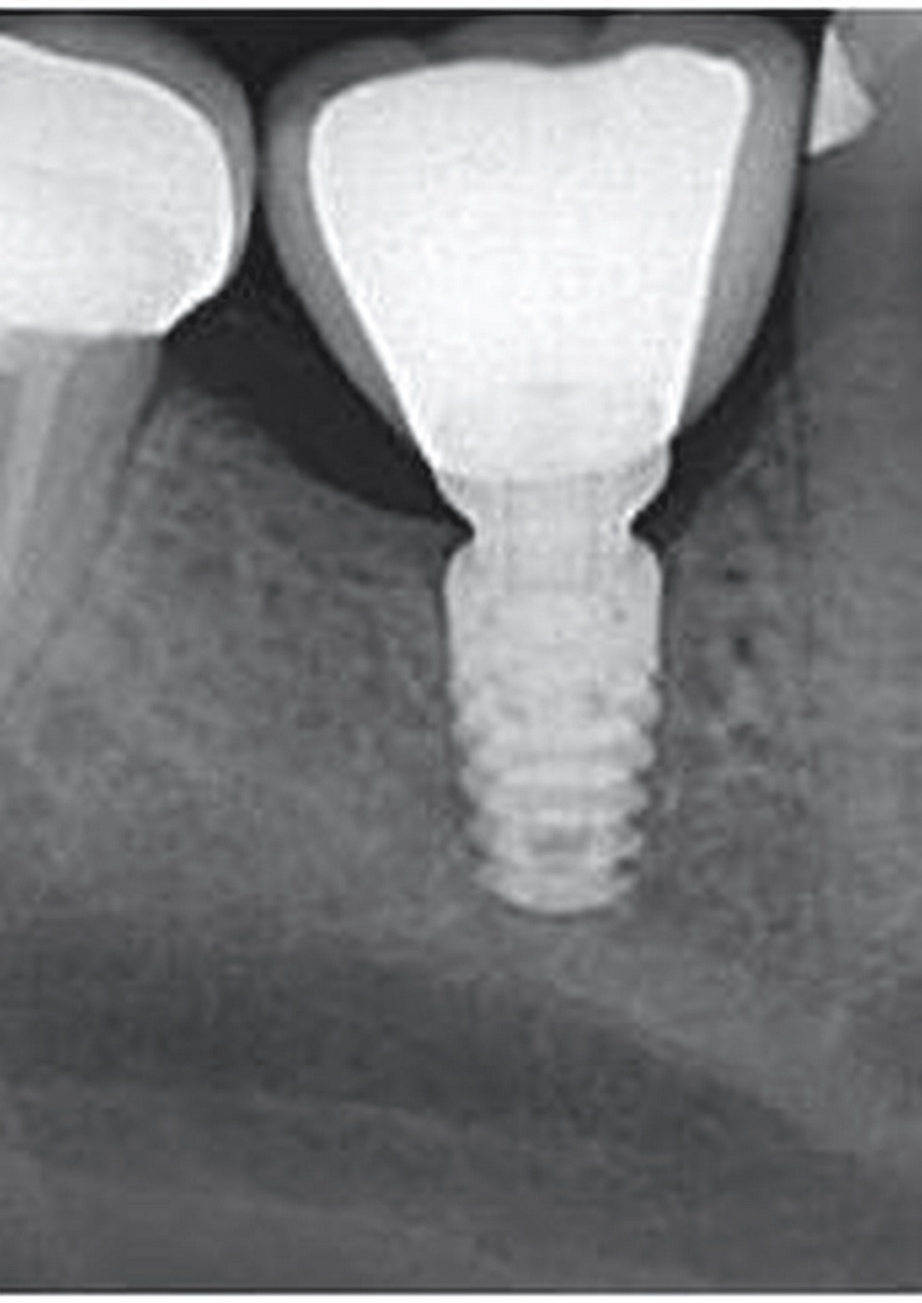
Radiographic image showing peri-implantitis
